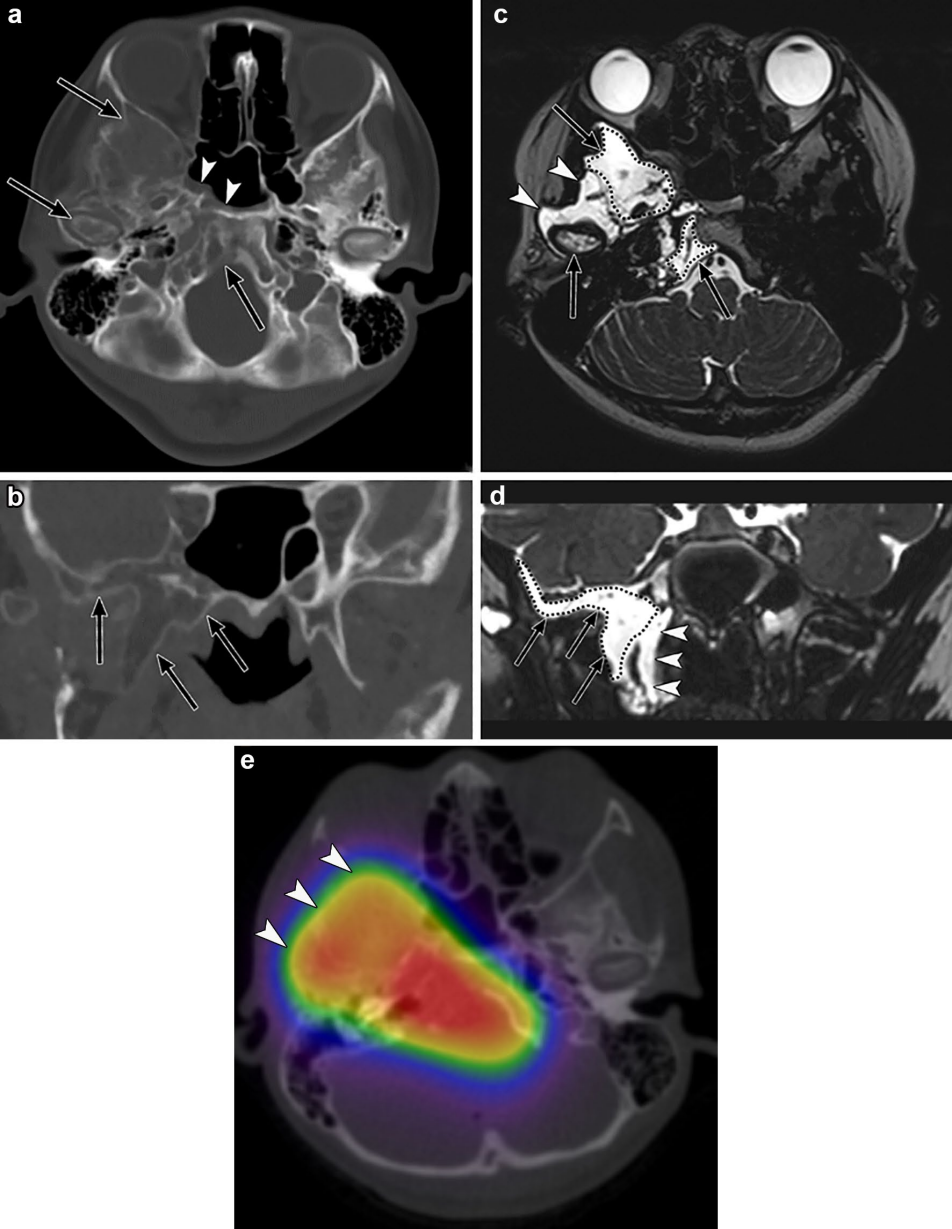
Gorham–Stout disease in a 12-year-old girl with recurrent bacterial meningitis. The chief complaint was right neck pain and trismus. a, b Axial and coronal head computed tomography (CT) (bone window) show infiltrative osteolytic changes and expansion of the bone in the right skull base bone. The sphenoid bone and the right mandibular condyle are involved (black arrows). Fluid accumulation is observed in the sphenoid sinus (arrowheads). c, d Axial and coronal head magnetic resonance images (T2-weighted images) show that the right skull base is extensively involved and appears hyperintense (black arrows). Fluid accumulation is observed in the parapharyngeal space, suggestive of a cerebrospinal fluid (CSF) leak (arrowheads). Note that it is difficult to distinguish involved bones from a CSF leak because both show similar high intensities on T2-weighted images. e Single-photon emission CT/CT fusion imaging in radionuclide cisternography shows radionuclide accumulation in the right cervical fascial space, and a CSF leak is confirmed (arrowheads)
